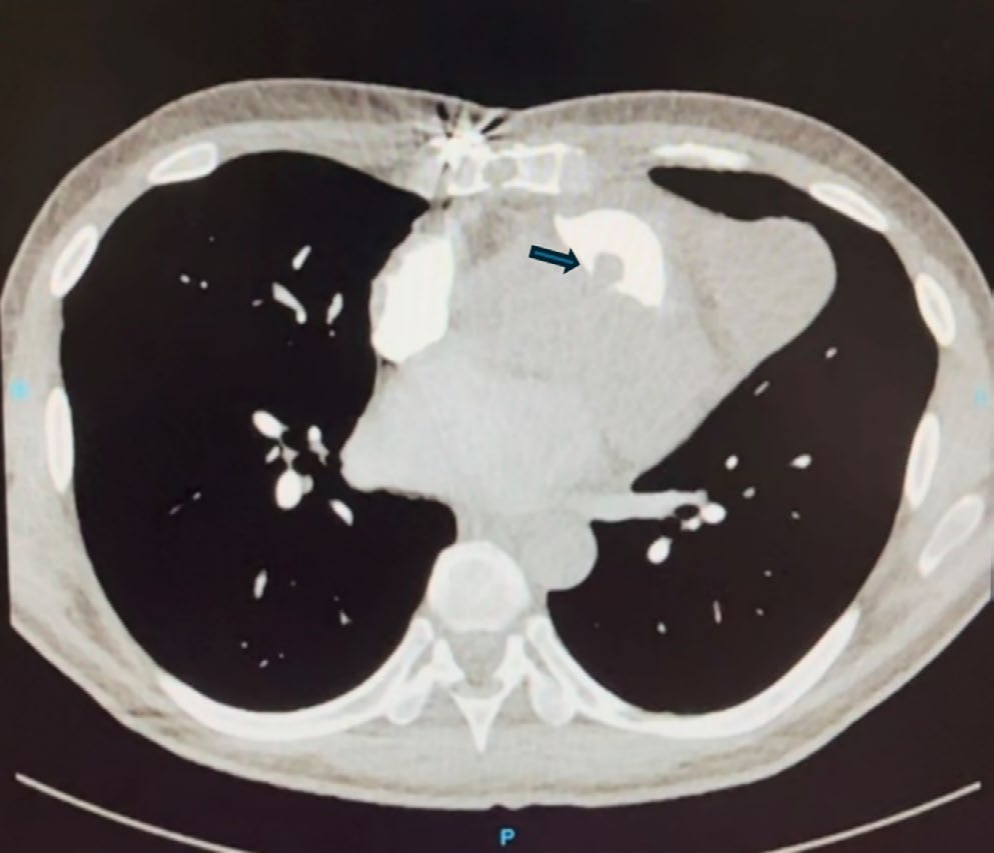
Computed tomography pulmonary angiogram showing a filling defect (15 × 10 mm) in RVOT suggestive of thrombosis (arrow). RVOT, right ventricular outlet tract.
